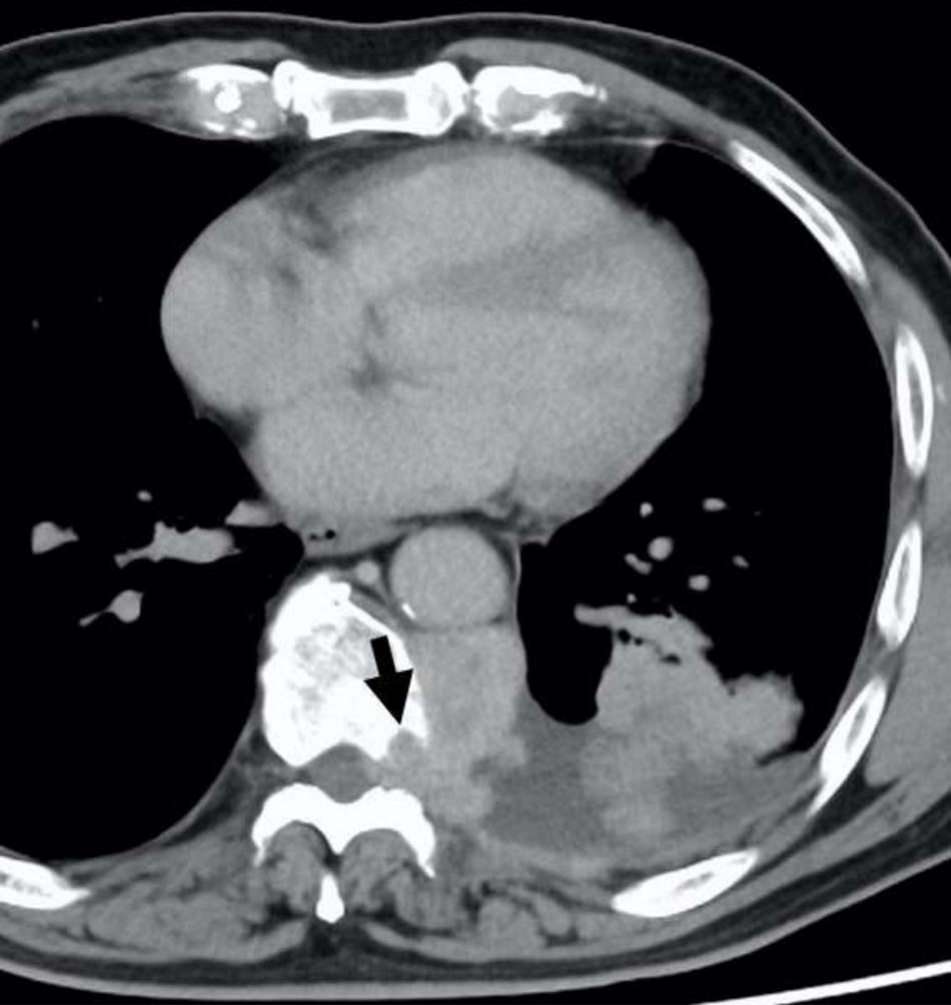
The computed tomographic findings of the current tumor; note the tumor extended through the neural foramen, and had an osteoclastic progression into the thoracic spine (arrow).
